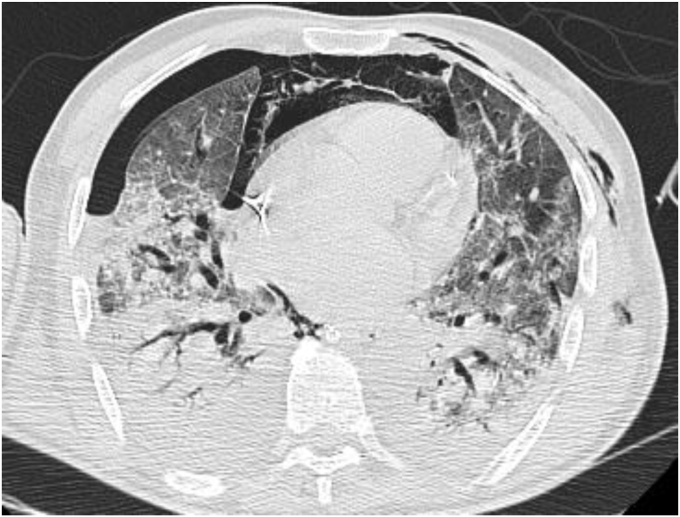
Axial chest CT image in a patient with severe advanced stage Covid-19 pneumonia complicated by right pneumothorax, pneumomediastinum and sub-cutaneous emphysema under mechanical ventilation.
